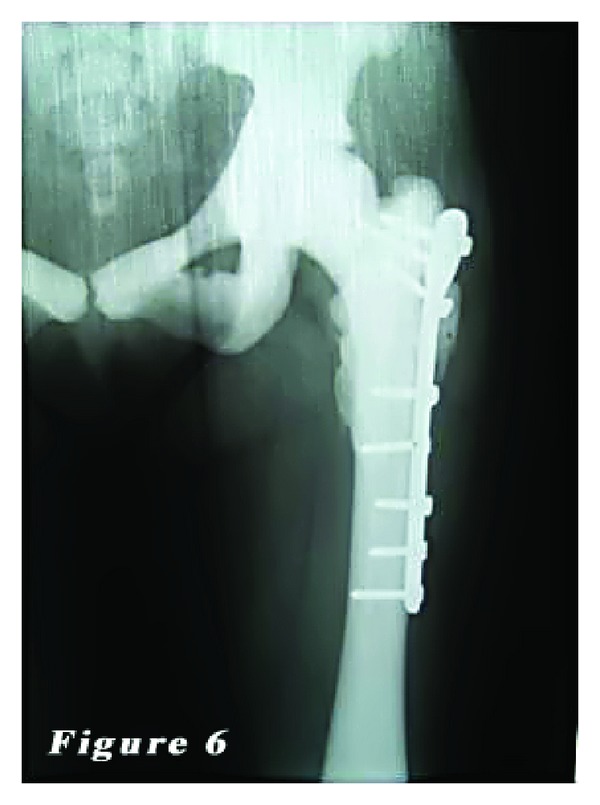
Her last left femur radiography. It looks like fracture union.
